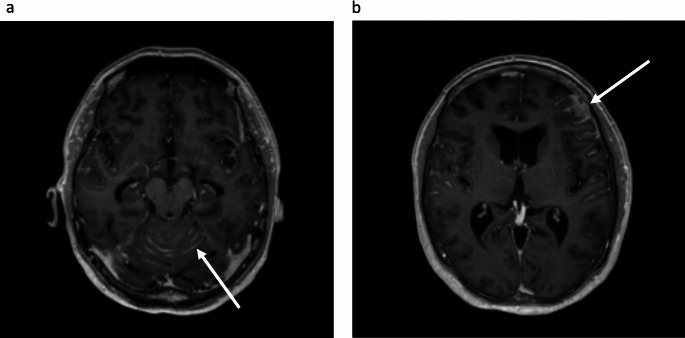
Magnetic resonance imaging of the brain. The images show extensive, abnormal contrast enhancement. The white arrows indicate meningeal contrast enhancement in the cerebellum (a) and left frontal sulci (b)
